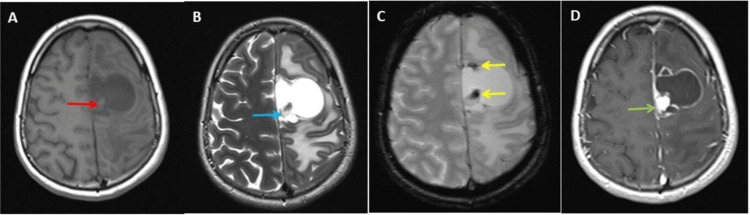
Magnetic resonance imaging (MRI) of the brain at a higher level showing the cyst and the hemoglobin (HEMO)-sensitive image of the cystic lesion and post-contrast images. The MRI of the brain at a higher level shows a small eccentric mural nodule in the cyst abutting the pia matter. This mural nodule isointense to white matter on T1-weighted image (panel A, red arrow), displays high T2 signals (panel B, blue arrow), and shows blooming/signal drop on the HEMO-sensitive image at the tip of the nodule and wall of the cyst (panel C, yellow arrows). Post-contrast T1 images display avid enhancement of the mural nodule abutting pia matter (panel D, green arrows).
